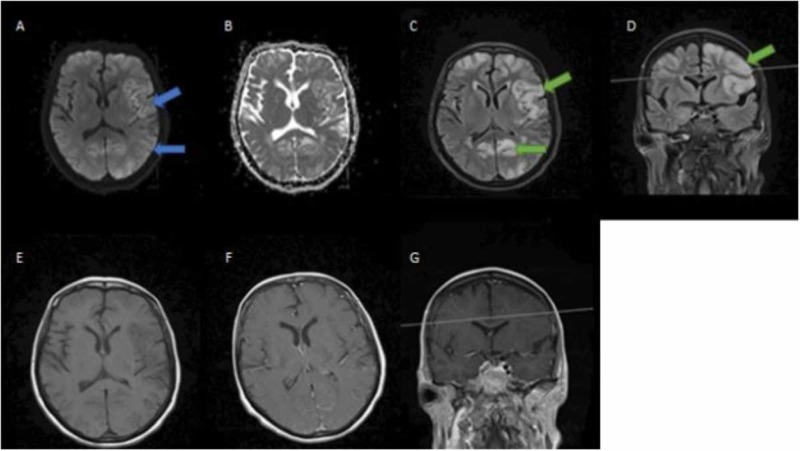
MRI of the brain with and without intravenous contrast A: axial DWI; B: axial ADC; C: axial T2 FLAIR; D: coronal T2 FLAIR; E: axial T1 FLAIR; F: axial T1 with contrast; G: coronal T1 with contrast There is an interval increase in the cortical and subcortical T2 FLAIR hyperintensities (green arrows), now involving bilateral cerebral cortex diffusely with no associated enhancement. There is new diffusion restriction in frontoparietal, temporal, insular, and occipital cortex on DWI sequences representing cortical ribboning (blue arrows), which is suggestive of CJD pattern MRI: magnetic resonance imaging; DWI: diffusion-weighted imaging; ADC: apparent diffusion coefficient; FLAIR: fluid-attenuated-inversion-recovery; CJD: Creutzfeldt-Jakob Disease
